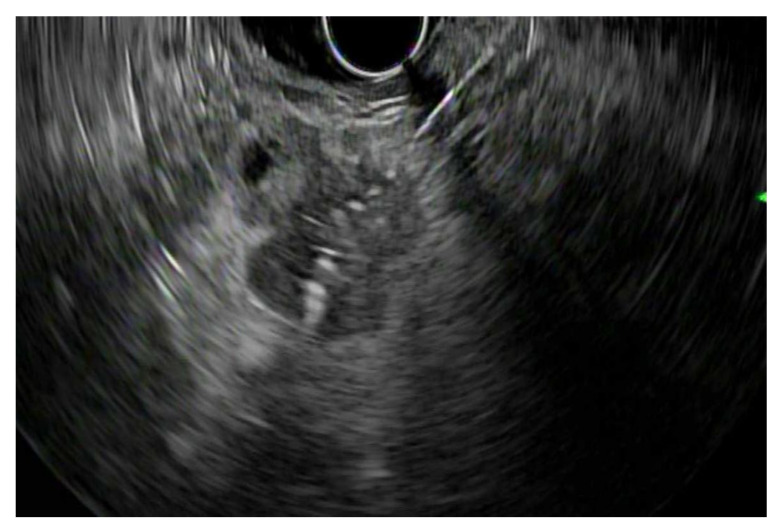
Endoscopic ultrasound-fine needle aspiration (EUS-FNA): Endosonographic image of an EUS-FNA of a pancreatic mass.
